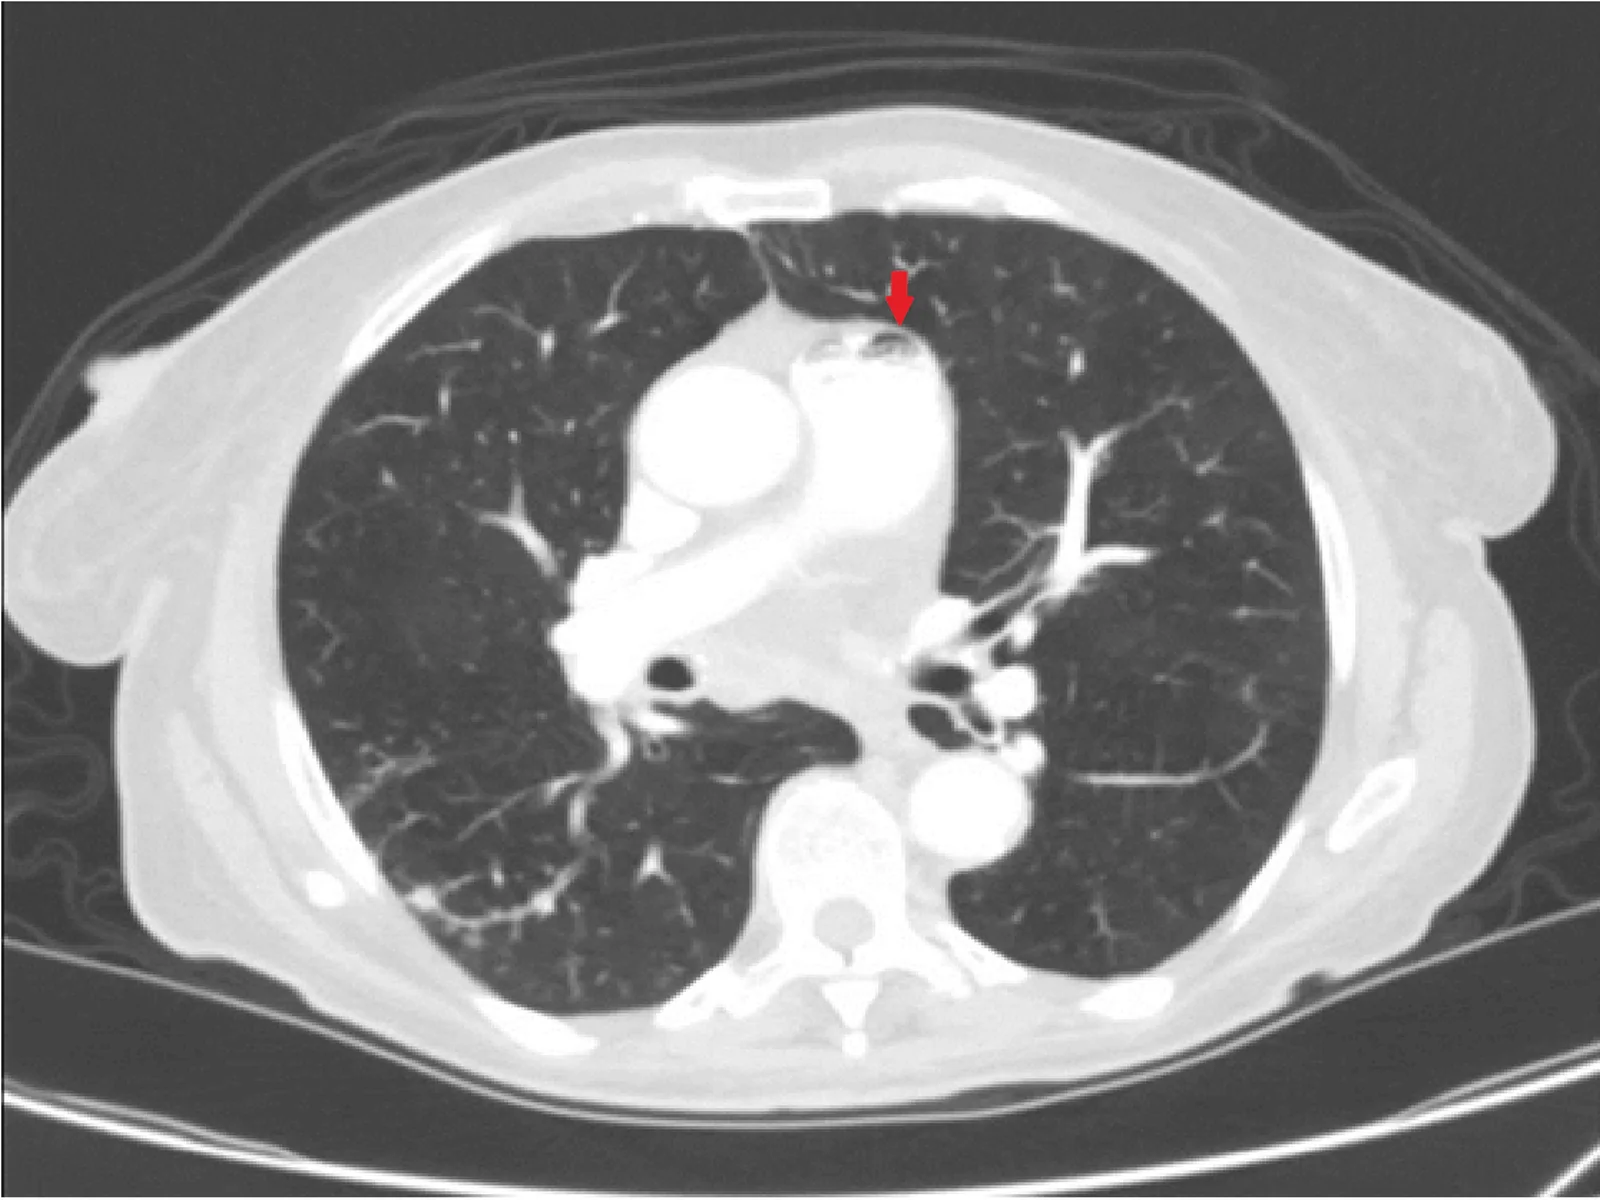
CT of the chest axial view Moderate air embolism within the main pulmonary artery and right ventricle, in the form of air bubbles of various sizes (red arrow). CT: computed tomography
